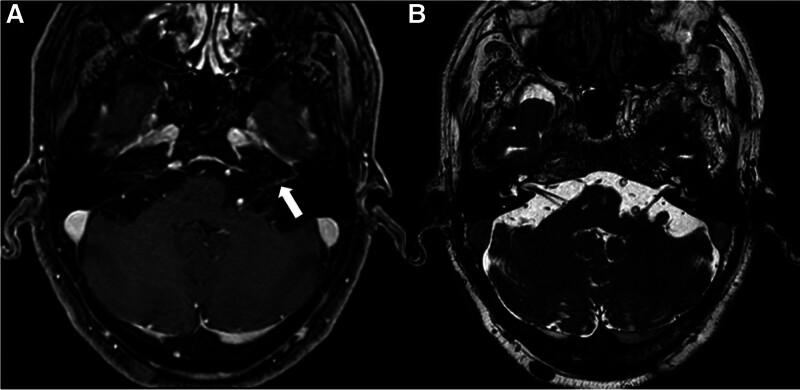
Contrast-enhanced MRI 3 months prior to presentation. Initial MRI with (*A*) contrast-enhanced T1 sequence and (*B*) heavily weighted T2 sequence of the CPA and IAC. Contrast-enhanced T1 sequence demonstrates mild enhancement of the left cochleovestibular nerve region (white arrow). No other appreciable retrocochlear pathology. CPA indicates cerebellopontine angle; IAC, internal auditory canal.
